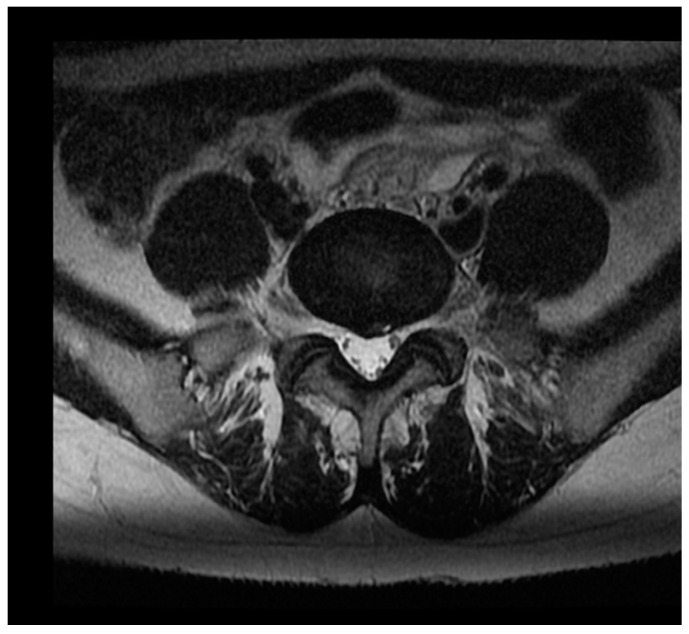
An example image of intervertebral disc protrusion in MRI (1.5 T, T2 transverse planes).
